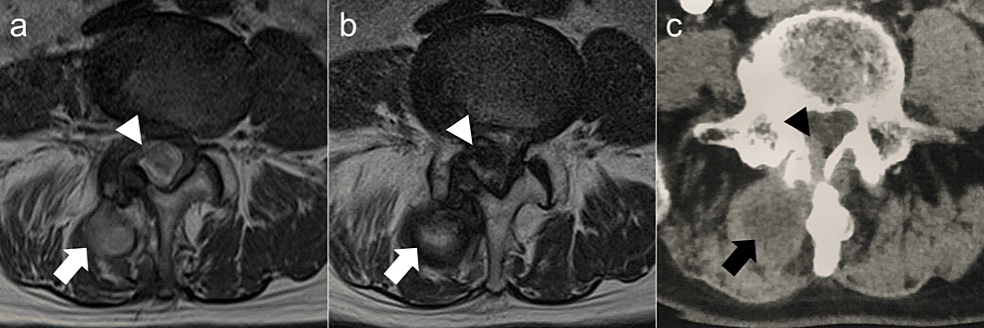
MRI and contrast-enhanced CT of intraspinal (arrowheads) and extraspinal space-occupying lesions (arrows) at the L4–L5 facet level on initial presentation. (a) Axial T1-weighted MRI shows an intraspinal, extradural SOL in the right dorsal aspect of the spinal canal and an extraspinal SOL in the multifidus. Both SOLs demonstrate isointensity to hyperintensity; (b) Axial T2-weighted MRI of these SOLs shows a partially hyperintense area surrounding a low-intensity area; (c) Contrast-enhanced CT of the intraspinal and extraspinal lesions at the L4–L5 facet level shows almost no enhancement. The sizes of the intraspinal and extraspinal SOLs are rostrocaudal 18 mm × dorsoventral 16 mm × mediolateral 30 mm and rostrocaudal 38 mm × dorsoventral 30 × mediolateral 30 mm, respectively. SOL: space-occupying lesion
